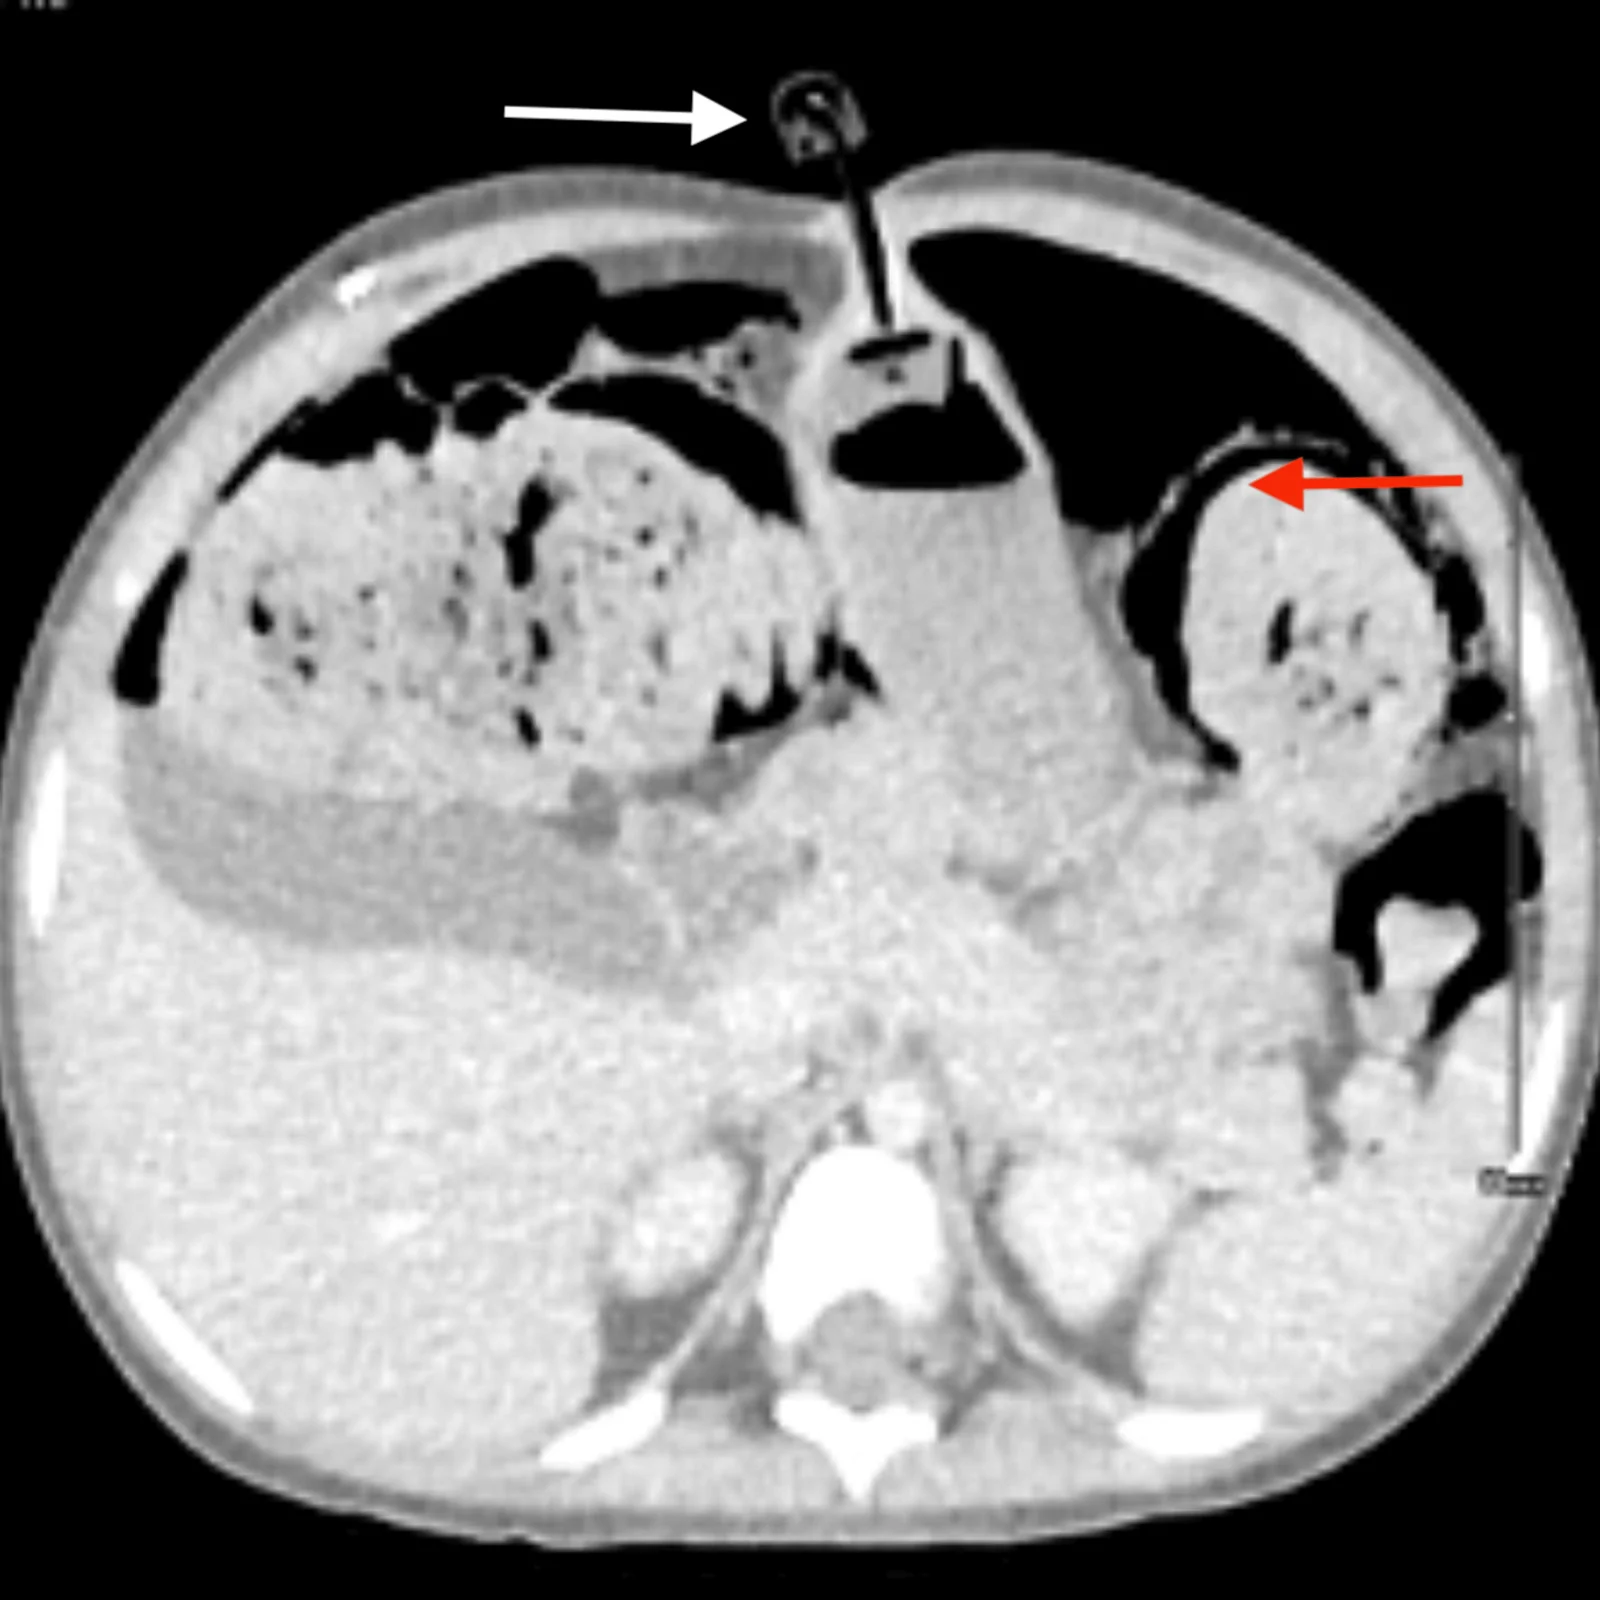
CT scan abdomen Axial image showing a large pneumoperitoneum, diffusely dilated loops of the small and large bowel filled with faeces, pneumatosis coli (red arrow), PEG tube in the stomach (white arrow) and no free fluid. PEG: percutaneous endoscopic gastrostomy
